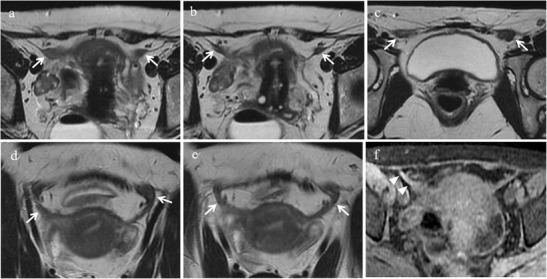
Endometriosis of the round ligaments in a 45-year-old woman with dysmenorrhea, urinary symptoms and chronic pelvic pain, who underwent a previous surgical intervention for endometrioma of the left ovary. (a-c) Oblique coronal and (d, e) oblique axial T2-weighted images. Both round ligaments (white arrows) appear thickened, nodular and shortened. (f) Oblique coronal fat-suppressed T1-weighted image reveals small intralesional high signal-intensity foci within the right ligament (white arrowheads) that represent hemorrhagic component
